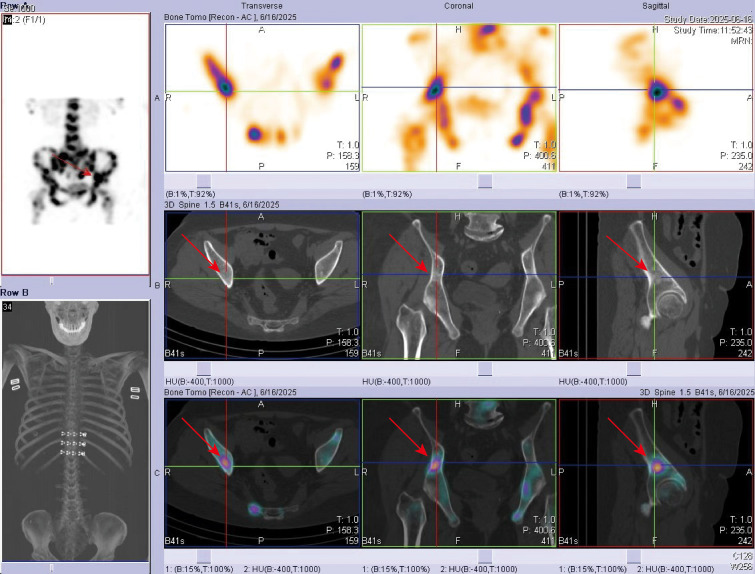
Single-photon emission computed tomography (SPECT/CT) of the pelvis. SPECT/CT demonstrated abnormal radiotracer uptake corresponding to patchy, mottled, and cotton-wool–like hyperdense lesions with marginal osteosclerotic changes in the iliac bone, supporting metastatic bone involvement. Red arrows indicate the representative pelvic lesion identified on axial, coronal, and sagittal images.
